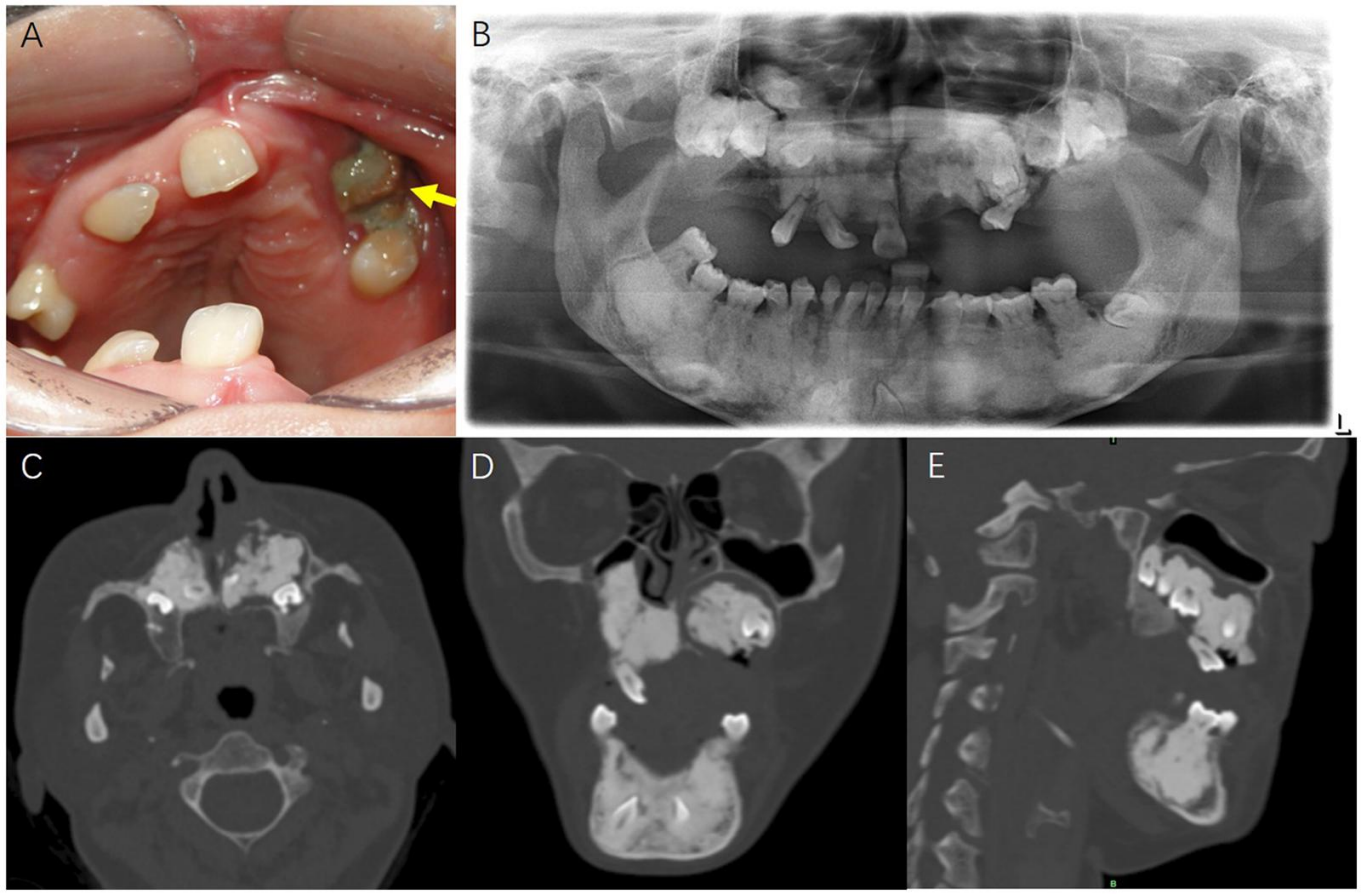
The representative intraoral photo and radiological images. (A) The intraoral image showed a 1.5 cm × 1.0 cm exposed bone (yellow arrow) in the left maxillary premolar region. (B) The pantomography showed diffuse, ill-defined radiopaque masses surrounding multiple impacted teeth in both maxilla and mandible. (C–E) The horizontal, coronal and sagittal CT images showed an extensive radiopaque mass in the left maxilla, surrounded by a radiolucent rim, with cortical dehiscence involved the nasal floor and buccal cortex.
